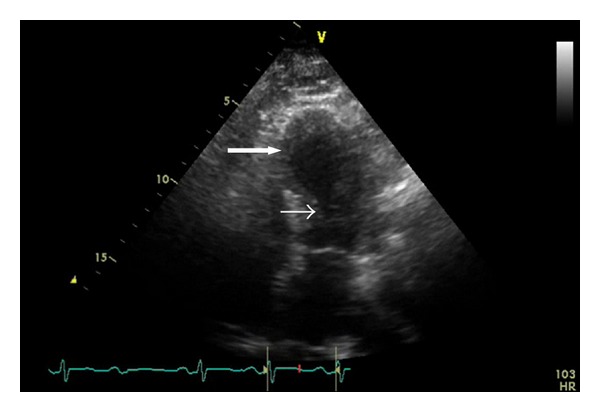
Transthoracic echocardiogram. The patient's apical four-chamber transthoracic echocardiogram at end systole showing left ventricle, left atrium, and mitral valve. There is left ventricular basilar contraction (small arrow), with near apposition of the endomyocardium, and severe apical hypokinesia (large arrow) with apical ballooning.
